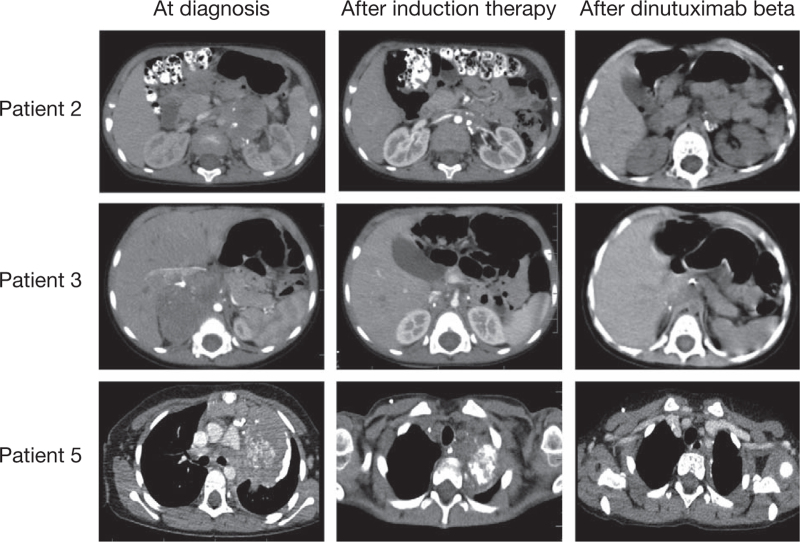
Abdominal/thorax CT scans at diagnosis (left panels), after induction therapy (middle panels) and after dinutuximab beta therapy (right panels) for patients who achieved a very good partial response with induction therapy: Patient 2, Patient 3, and Patient 5. CT = computed tomography.
